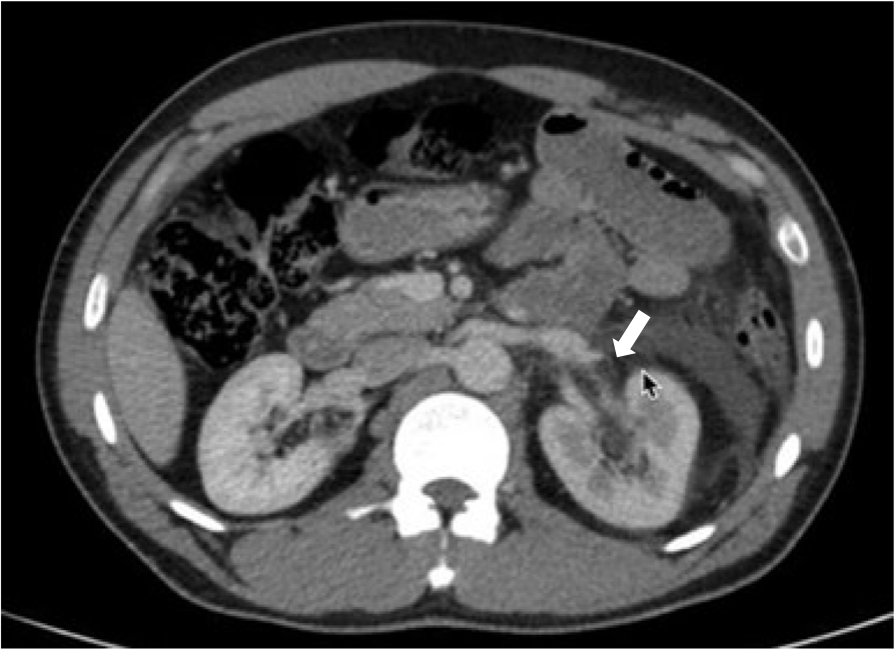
Abdominopelvic CT scan with contrast showing perinephric fluid and renal pelvis rupture
